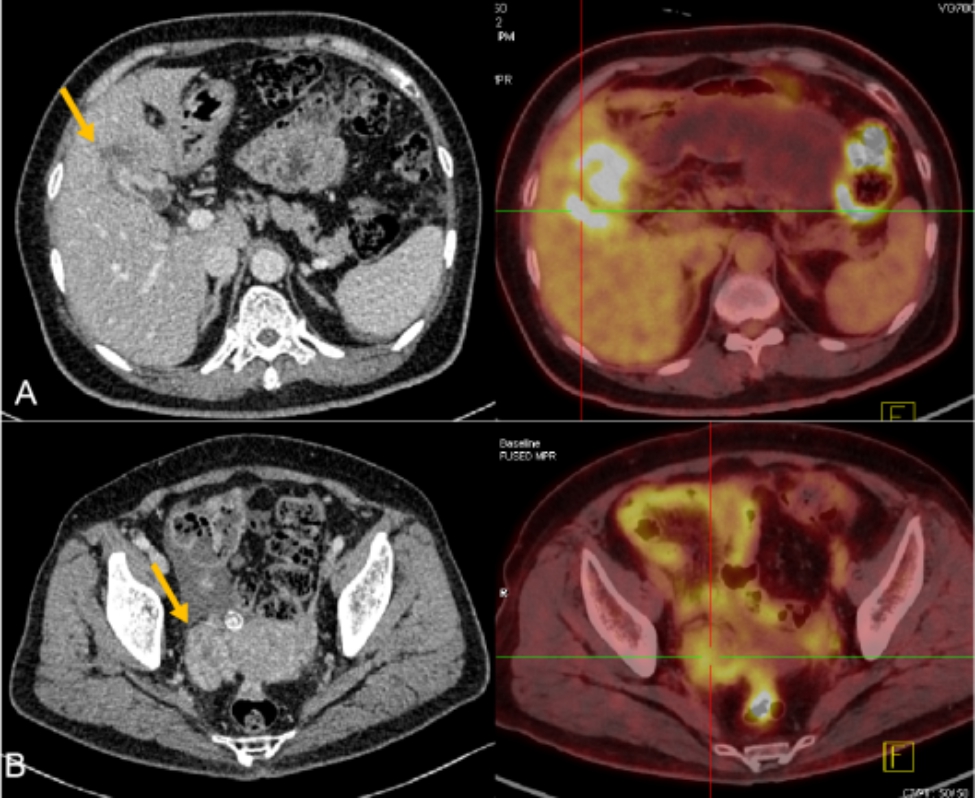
Computed tomography (CT) and positron emission tomography (PET)-CT imaging. (A) PET-CT showed increased fluorodeoxyglucose (FDG) uptake in the fossa of the gallbladder and adjacent liver tissue, with a maximum standardized uptake value (SUV) of 8.2. CT showed enhancement of the thickened gallbladder wall and a hypodense lesion in the liver with ill-defined boundaries. (B) PET showed increased FDG uptake in the right adnexal area with a maximum SUV of 5.8, and CT showed soft tissue nodules
